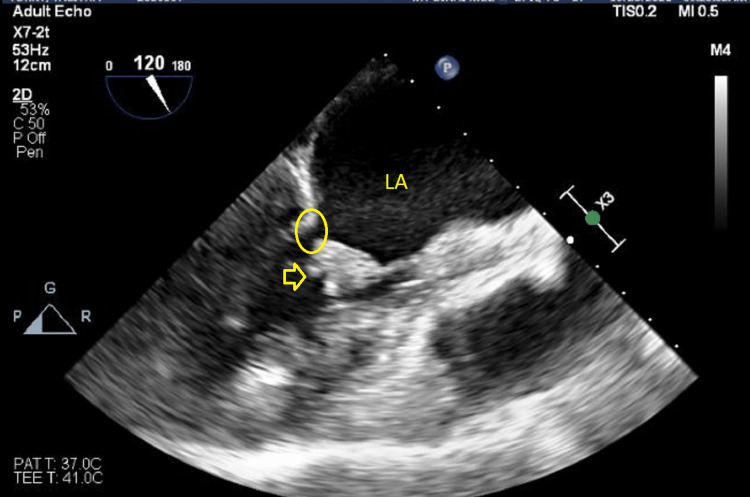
Transesophageal echocardiography showing infectious endocarditis with vegetations on the leaflets (yellow arrow) and severe mitral stenosis (yellow circle) in the setting of chronic rheumatic valve disease
